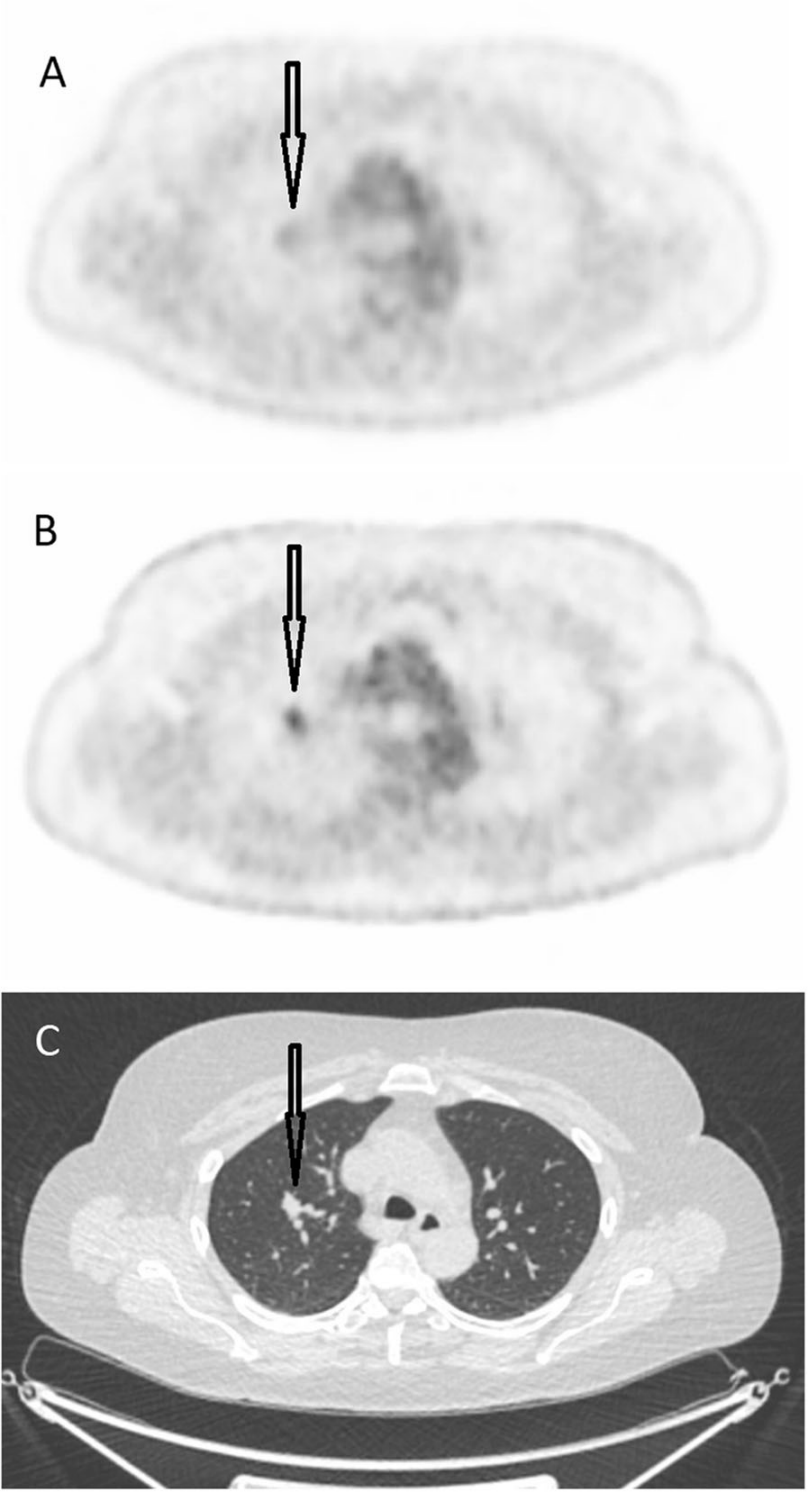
a) A transversal image from the Gemini TF. b) The same image from the Discovery MI and c) the corresponding CT image. The arrow indicates a tumour in the right lung, which was interpreted as malignant in b
